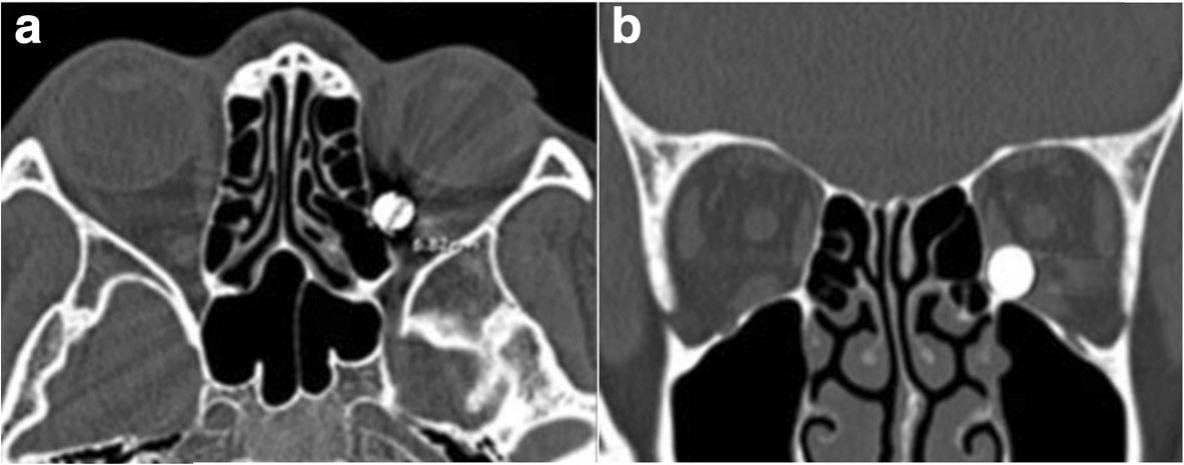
An axial (a) and coronal (b) cut section of a computed tomography scan image of the left orbit shows a round-shaped metallic foreign body embedded in the medial intraconal space lying lateral to posterior ethmoid sinus
